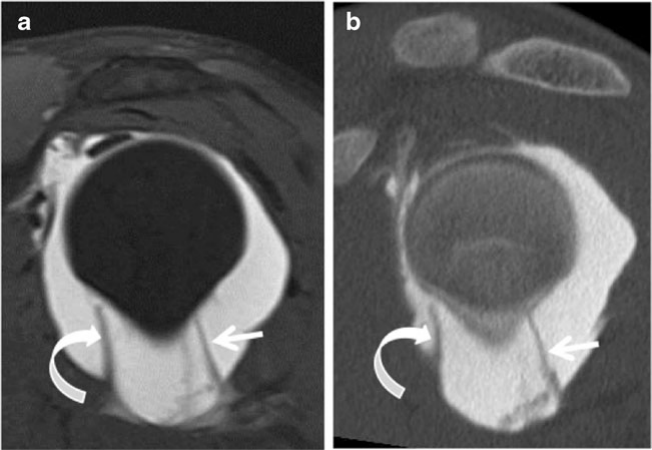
Inferior glenohumeral ligament. Sagittal (a) fat-suppressed T1-weighted MR-arthrography and (b) corresponding CT - arthrography images show the anterior band (*curved arrow*) and posterior band (*straight arrow*) of the inferior glenohumeral ligament
